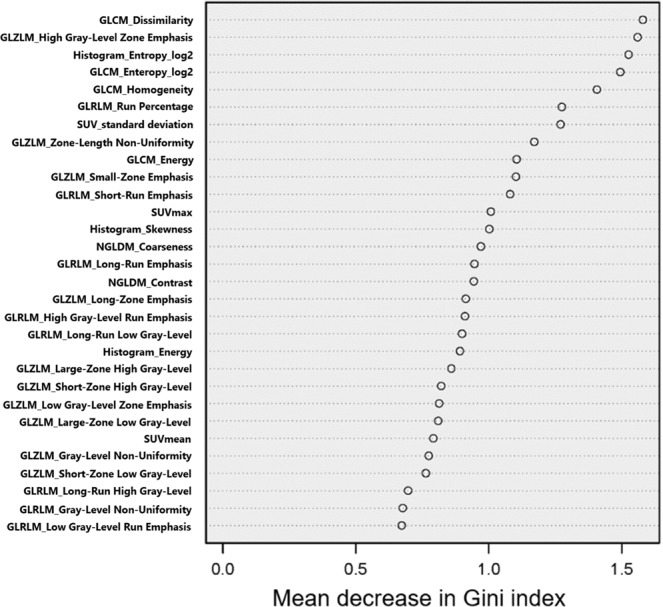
Mean decrease in Gini index showing the importance of PET parameters for the classification between recurrent brain tumor and radiation necrosis in the random forest classifier. GLCM = gray-level co-occurrence matrix; GLRLM = gray-level run length matrix; GLZLM = gray-level zone length matrix; NGLDM = neighborhood gray-level different matrix; SUV = standardized uptake value.
